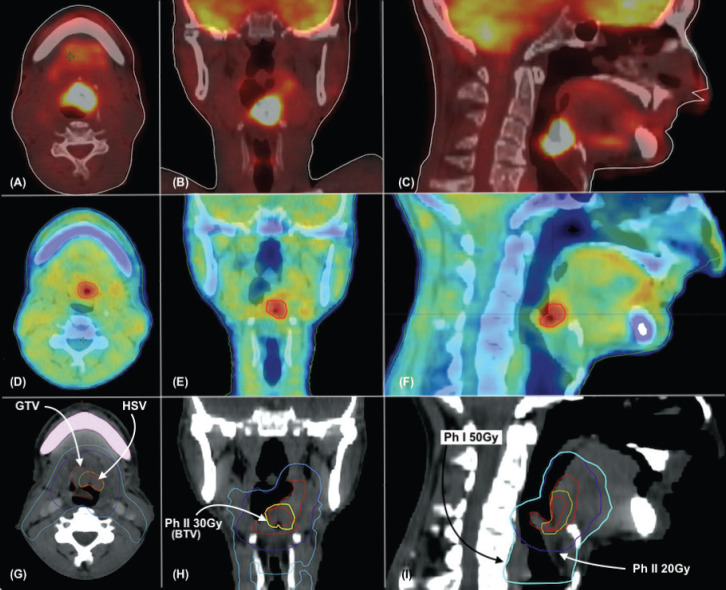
(a–c) shows 18F FDG PET CT images in axial, coronal and sagittal views, respectively. (d–f) shows 18F FMISO PET CT images in axial, coronal and sagittal views, respectively. (g–i), target delineation as labeled in axial, coronal and sagittal views, respectively. GTV – Gross Tumour Volume, HSV – Hypoxic Sub-Volume, Ph I 50 Gy – Volume receiving 50 Gy in 25 fractions in phase 1, Ph II 20Gy – Volume receiving 20 Gy in 10 fractions in phase 2. Ph II 30Gy – Volume receiving 30 Gy in 10 fractions in phase 2.
